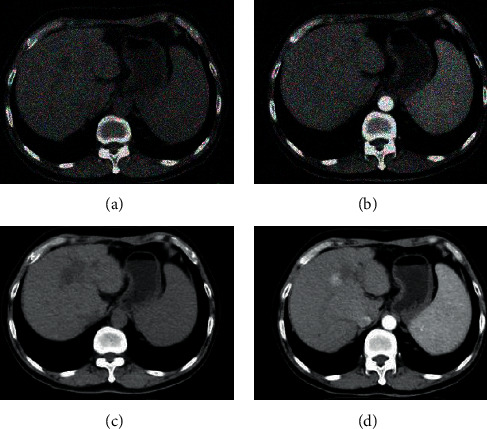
Comparison of CT images before and after surgery. (a, b) Preoperative CT images; (c, d) postoperative CT images processed by intelligent algorithms.
